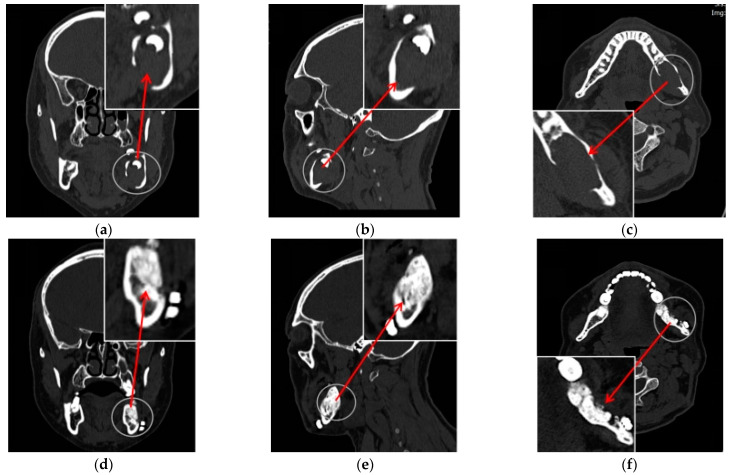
A case of jaw cysts treated with HBOT as adjunctive therapy. (a–c) Show the preoperative computed tomography (CT) images of the patient’s cysts; (d–f) show the six-month postoperative CT images after surgery.
